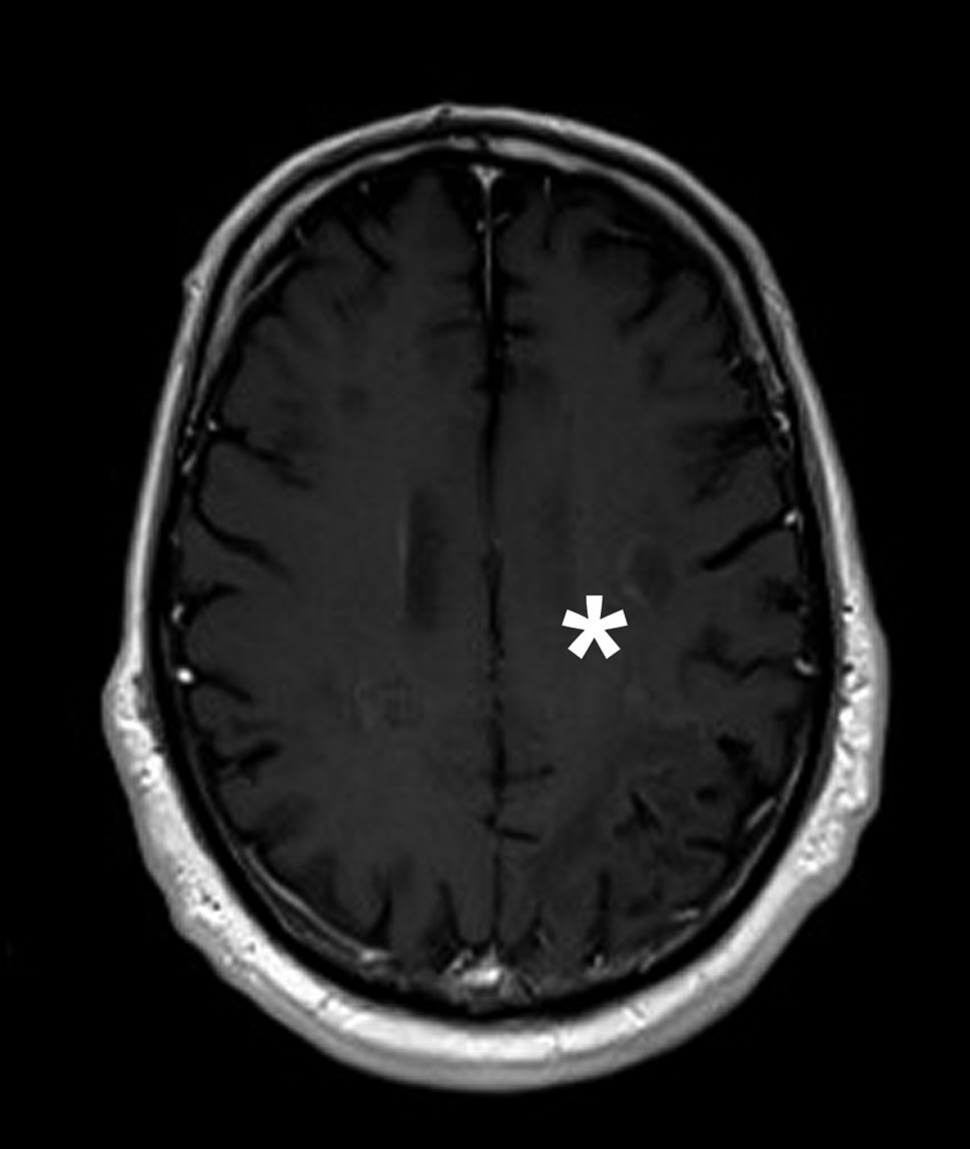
MRI of the head from January 2020 showing contrast enhancement. Shown is a T1 postcontrast MRI scan from January 2020. Slight gadolinium enhancement is marked with an asterisk (*)
